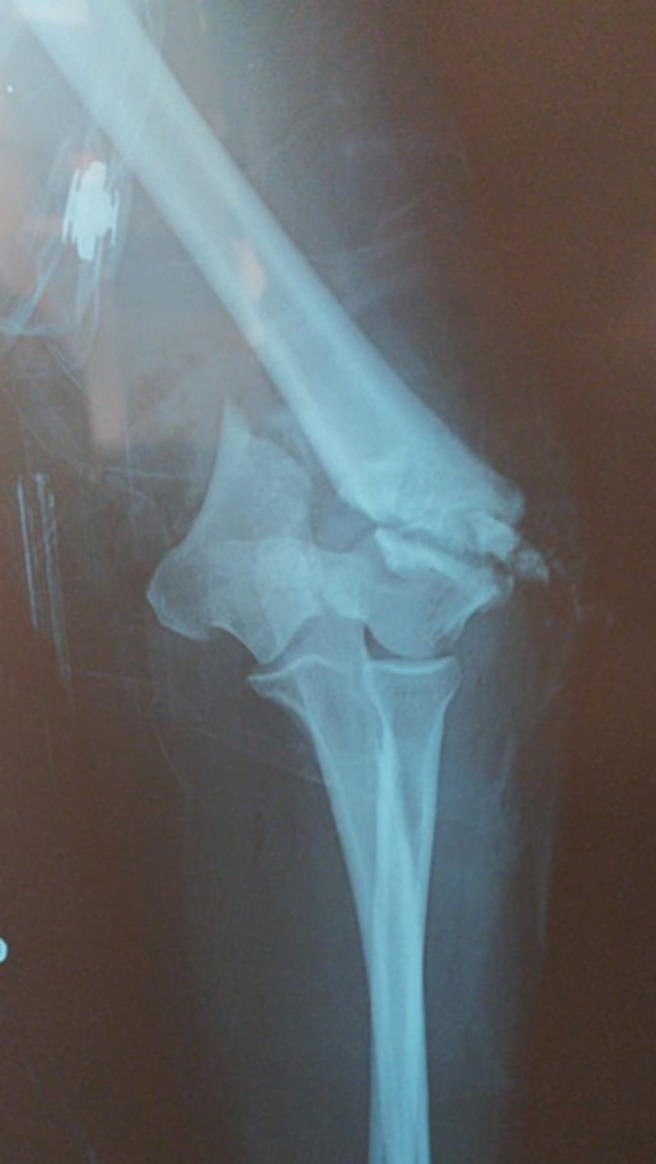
radiographie de face du coude objectivant une fracture sus et inter-condylienne de l’extrémité inférieure de l’humérus classée type C2 selon l’AO
